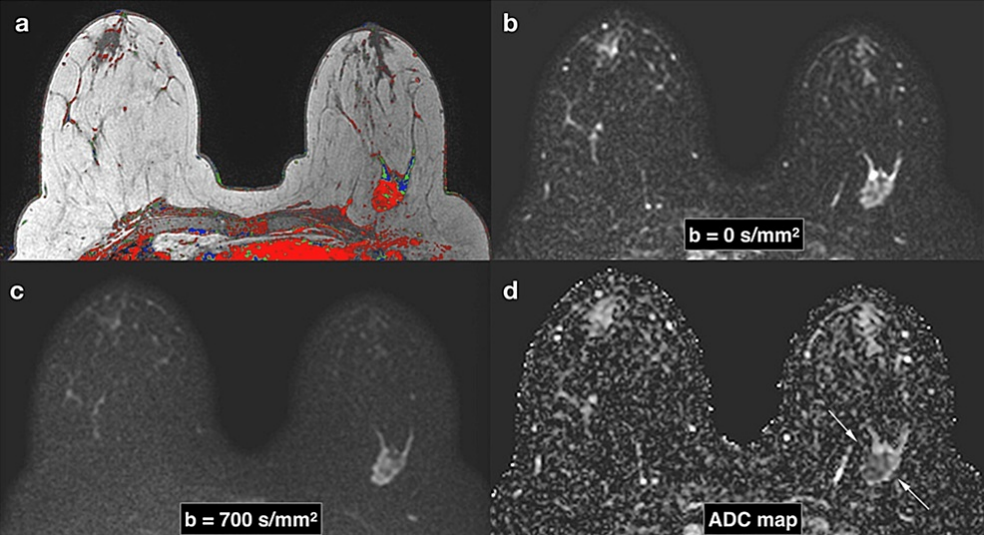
Triple-negative subtype left breast cancer shown on MRI with diffusion-weighted sequencing with corresponding ADC map Panel A shows a dynamic contrast-enhanced MRI post-contrast highlighting a posterior left breast cancer. Shown in Panel B is the MRI with diffusion-weighted sequencing at b=0 s/mm2, where B reflects the degree of diffusion weighting applied. Panel C shows the same breasts at b=700 s/mm2, which does improve the emphasis placed on the suspicious lesion. Both Panels B and C demonstrate that malignant lesions are hyperintense on DWI. Panel D corresponds to the ADC map in which the left breast cancer appears hypointense. *Source*: Camps-Herrero J. [17] with permission of image used under Creative Commons License: CC BY 4.0.
